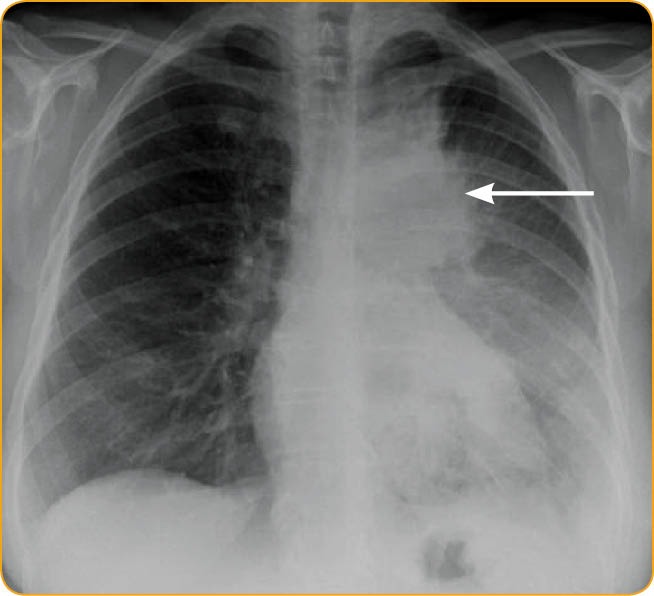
Chest x-ray shows a mass in the left upper lobe (arrow).
